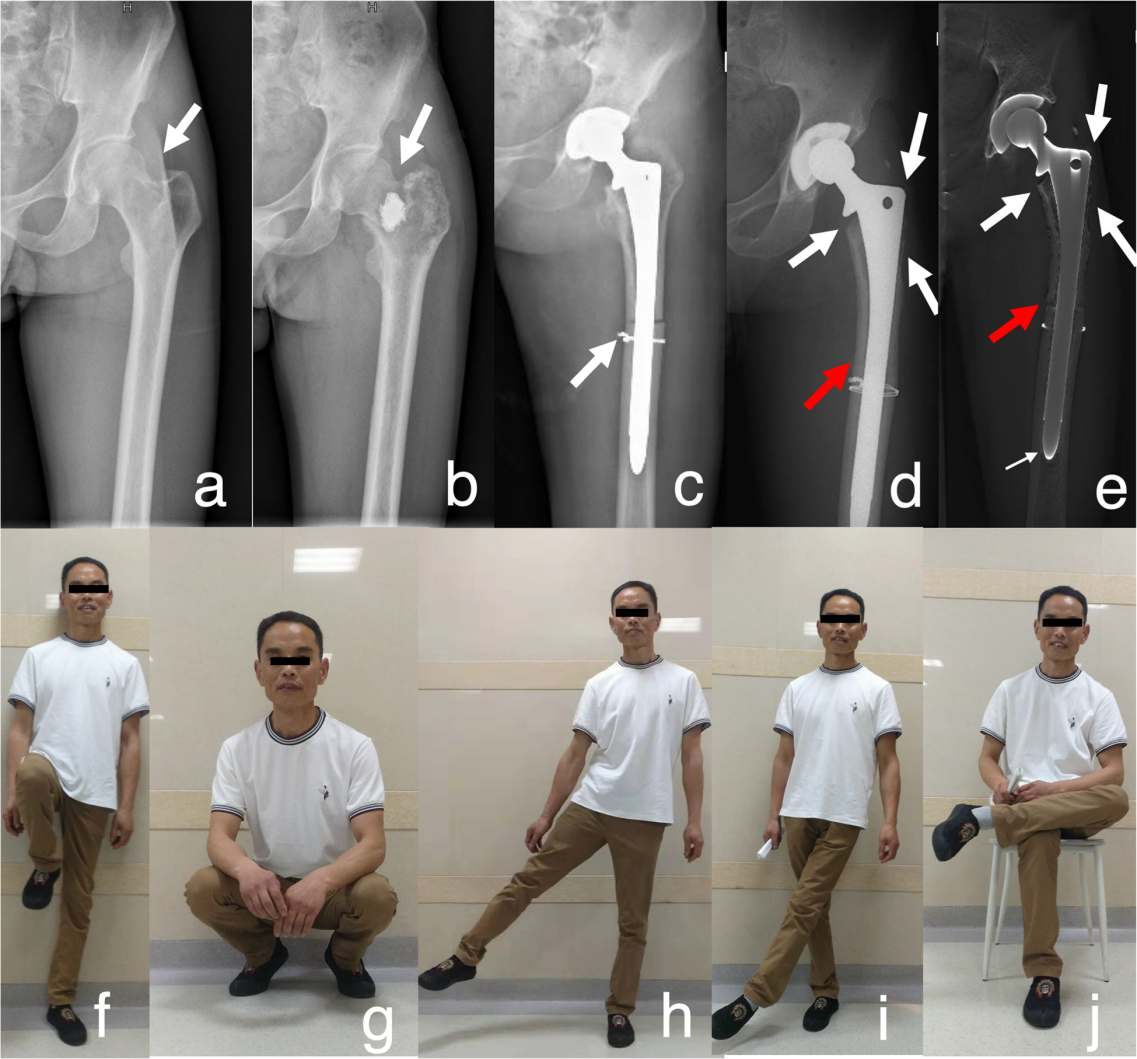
Patient (No.10) with the diagnosis of OS of the left femoral neck. a The white arrow indicated the lesion. b The white arrow indicated the pathological fracture occurred after the incisional biopsy during chemotherapy. c Immediate radiograph after the surgery of uncemented APC, the white arrow expressed intraoperative fracture and fixed with cerclage wires. d-e The last follow up radiograph and Tomosynthesis-Shimadzu metal artifact reduction technology (T-SMART) 107 months post-operation: the thick white arrows showed the resorption of the Gruen 1, Gruen2 and Gruen 7, the red arrows indicated bone union at the junction, the thin white arrows showed bone growing around the distal stem. (f-j) The function of the hip, 107 months post-operation
